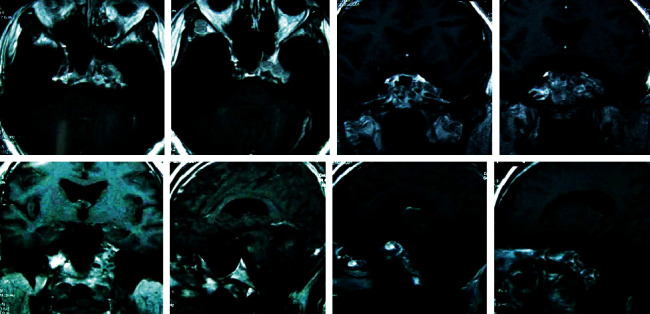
Conventional MRI scanning image of a patient with nasopharyngeal carcinoma.
